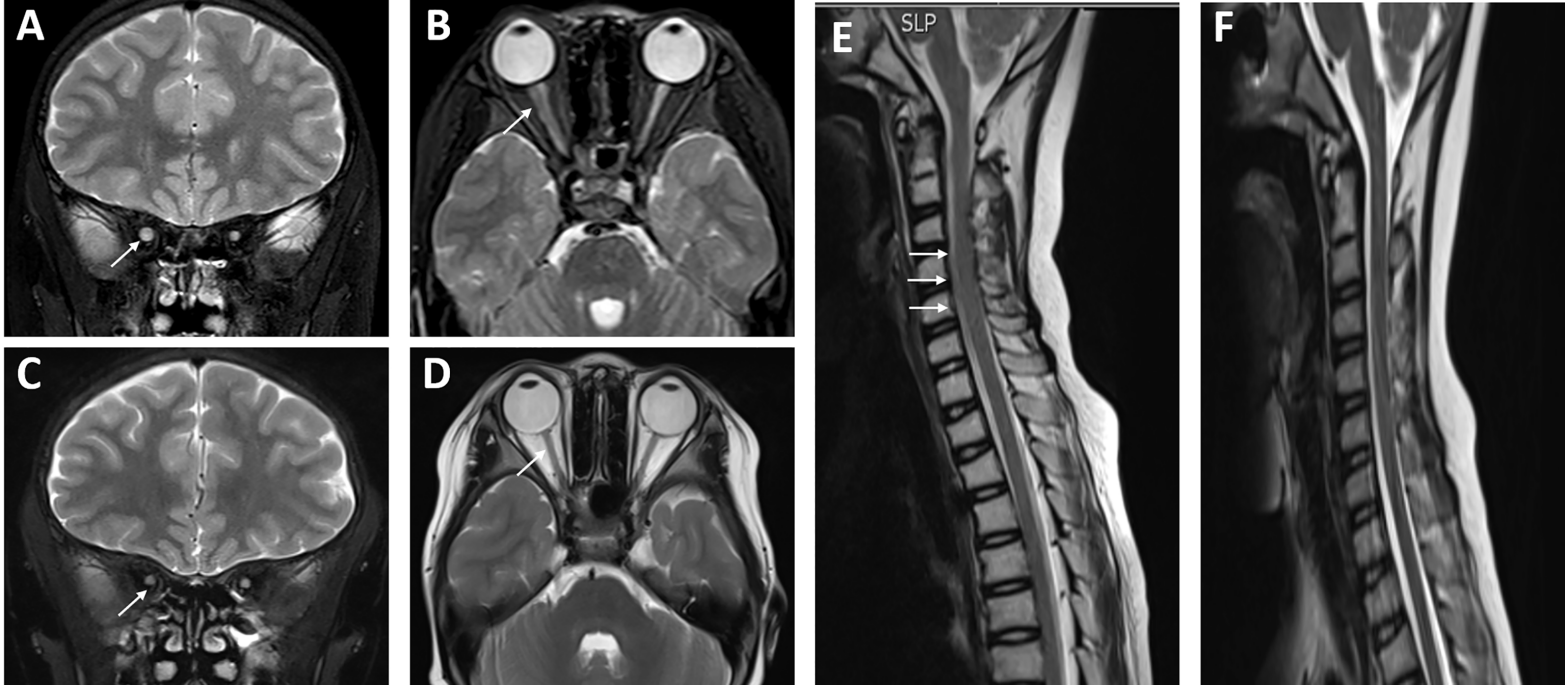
Neuroimaging changes of the patient with systemic lupus erythematosus (SLE)/Sjogren’s syndrome (SS) and NMOSD. (A, B) The left optic nerve was obviously smaller than the right through MRI, and there was slight swelling of the spinal cord at the levels of c2-6 (E) in December 2018 (shown by arrows). After a powerful immunosuppressive therapy, the left optic nerve was roughly the same size as the right optic nerve in October 2019 (C, D), and the swelling of c2-6 was much better than before (F).
